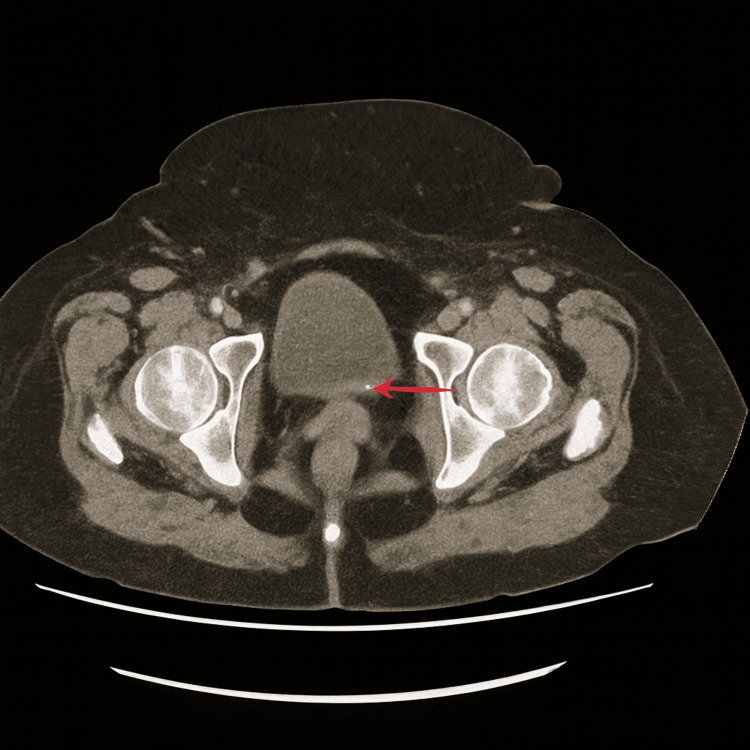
Axial view of CT abdomen and pelvis showing a stone at the left vesicoureteric junction (red arrow).
